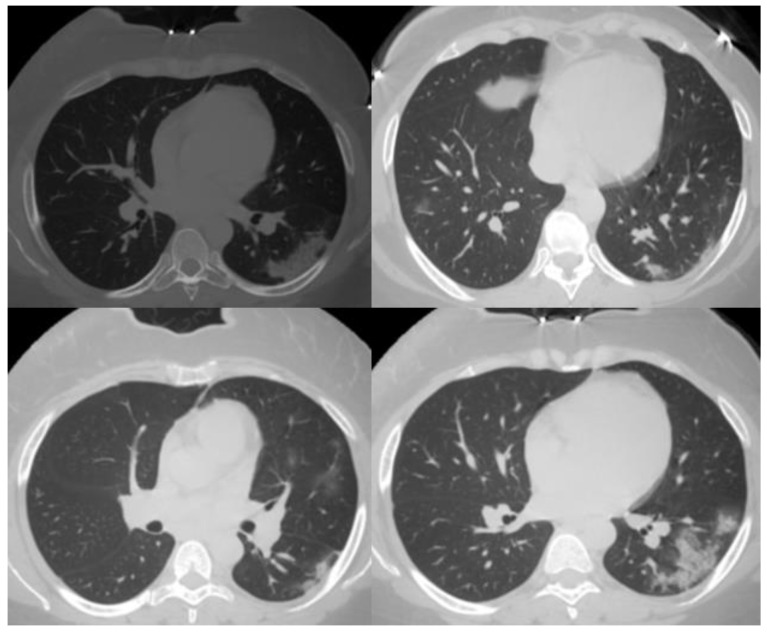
Multiple chest CT images of COVID-19 patients. Senior radiologists and the pulmonologist agreed to calculate the CO-RADS for this case as CO-RADS 4. The agreement was based on the following features: typical features of multifocal unilateral GGO, consolidation, unifocal GGO on the other side, organizing pneumonia without typical features, multiple unilateral ventrilobular GGO, and focal unilateral GGO. The junior radiologist and house officers agreed to calculate the CO-RADS for this patient as CO-RADS 5. The agreement was based on the following features: multifocal bilateral GGO and consolidation with organizing pneumonia.
